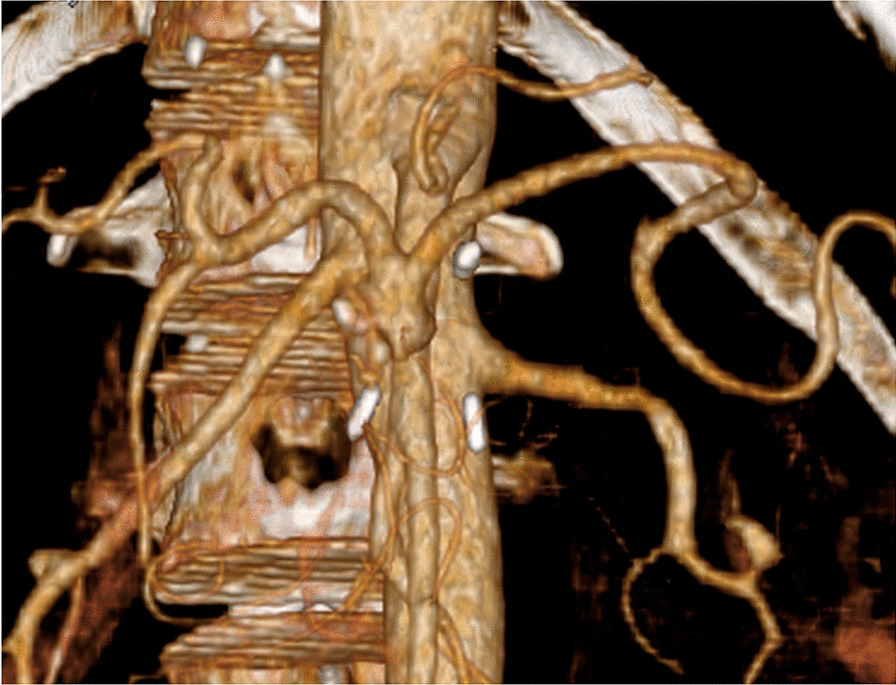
Postoperative enhanced computed tomography findings. The celiac artery is anatomically reconstructed after celiac artery aneurysm resection
